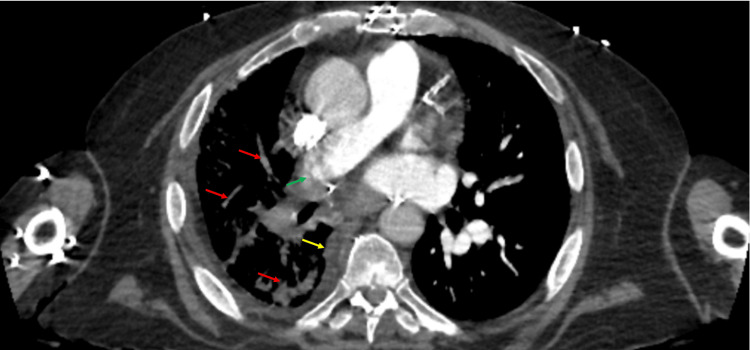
Computed tomography scan of the chest with contrast demonstrating reticular and patchy consolidative opacities in the right lung (red arrows), pleural thickening at the right lung base (yellow arrow), and high-grade stenosis in the distal right main pulmonary artery (green arrow).
